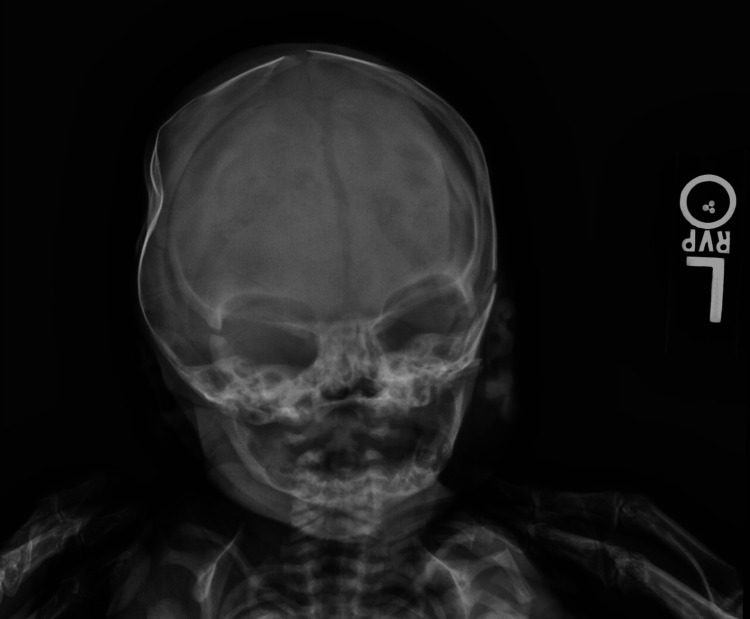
Head X-ray of the neonate revealing concave deformity of the right parietal calvarium with concomitant diastasis of the sagittal suture.
